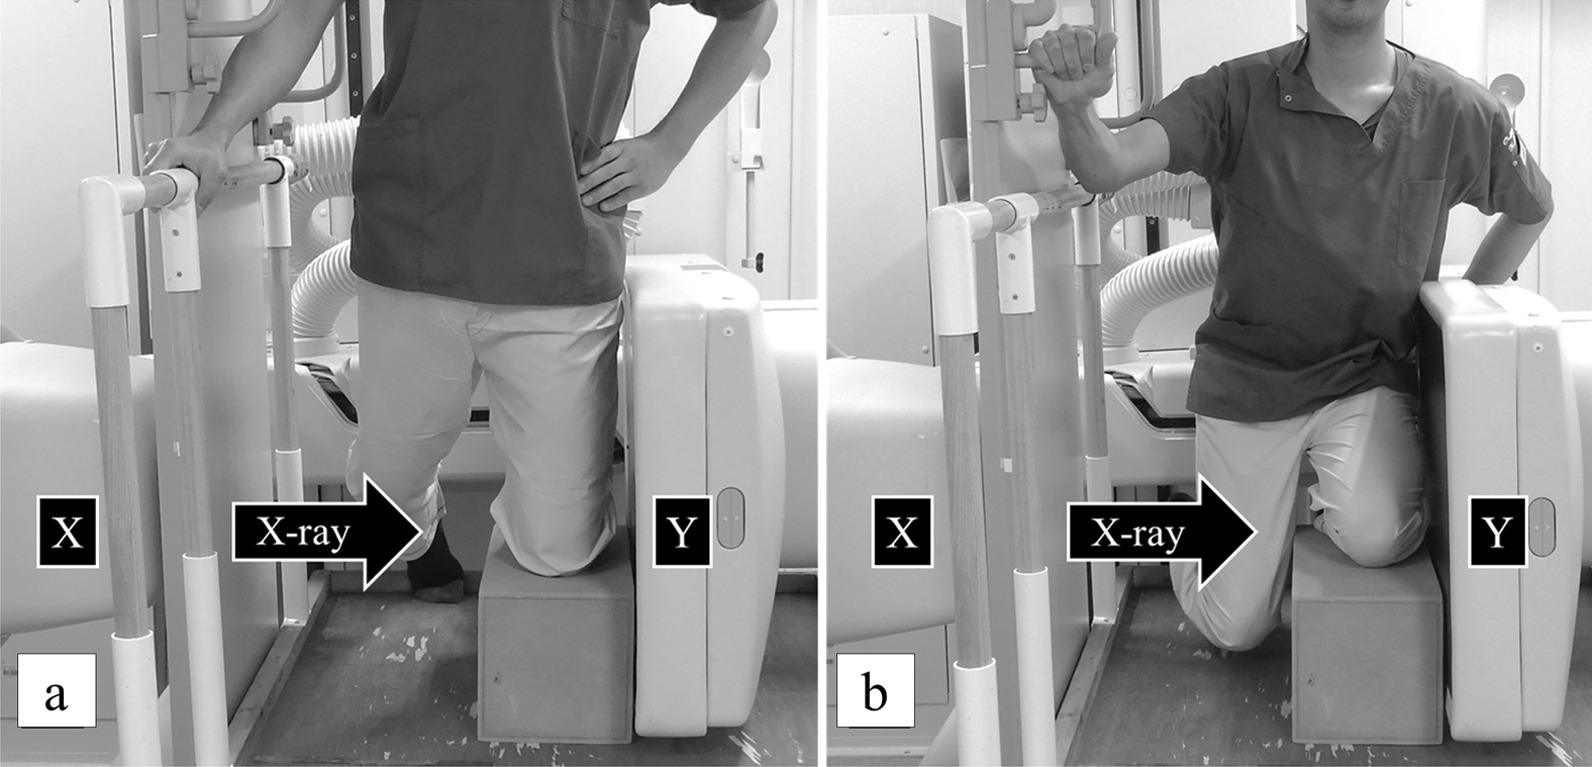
Kneeling action and lateral fluoroscopic imaging. a Represents the starting posture when the subject is kneeling with a knee flexion angle of 90°. b Represents the posture when the subject flexes the knee to the maximum flexed angle from (a). X-rays are emitted from the radiation source (X) toward the flat panel (Y), and a series of actions are recorded
